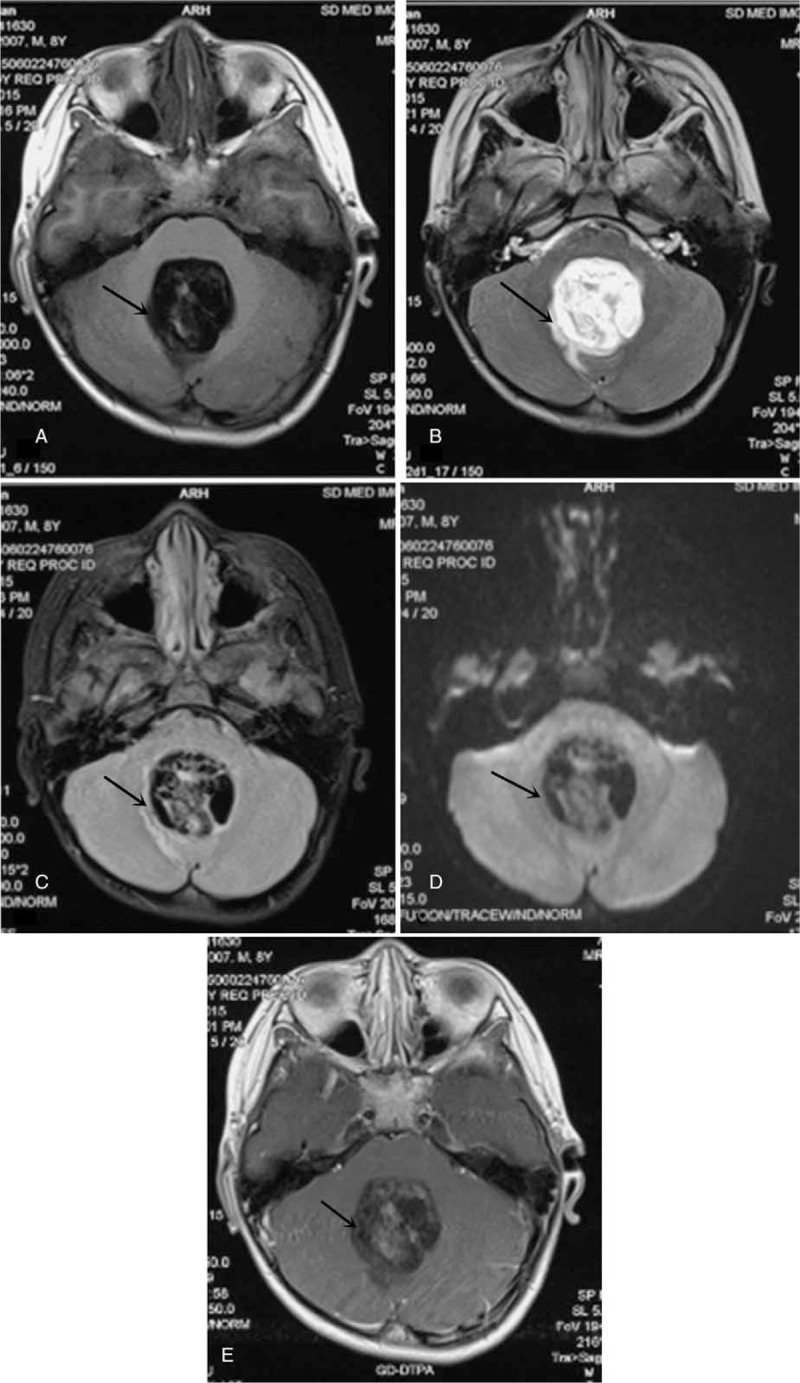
Preoperation MRI (A) T1-weighted sequences. (B) T2-weighted sequences. (C) DWI (*b* = 1000). (D) FLAIR sequences. (E) Image after Gd-DTPA injection. MRI = magnetic resonance imaging, DWI = diffusion weighted imaging, FLAIR = fluid attenuated inversion recovery, Gd-DTPA = gadolinium-diethylenetriamine pentaacetic.
